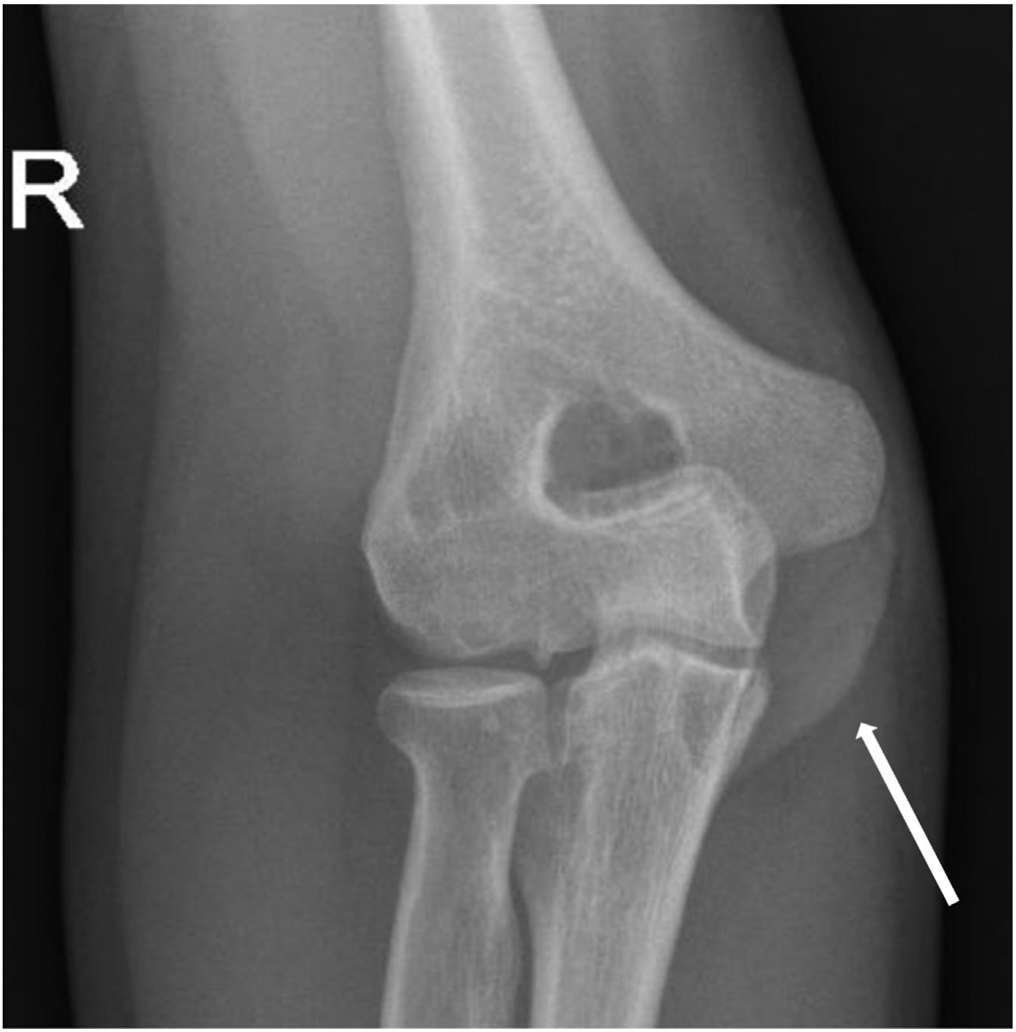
Radiograph showing a soft tissue mass (arrow) on the right elbow, without any evident of bone erosion.
